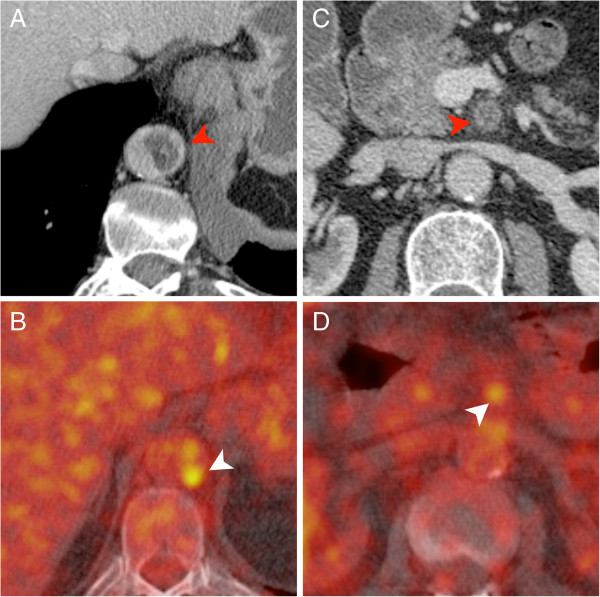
MDCT and PET/CT of a 71-year-old male patient with an undifferentiated intimal sarcoma of the descending aorta and extension into the superior mesenteric artery (A, C). The PET/CT adds valuable information about the metabolic status of vascular filling defect and strengthens the suspicion of malignant disease (B, D).
